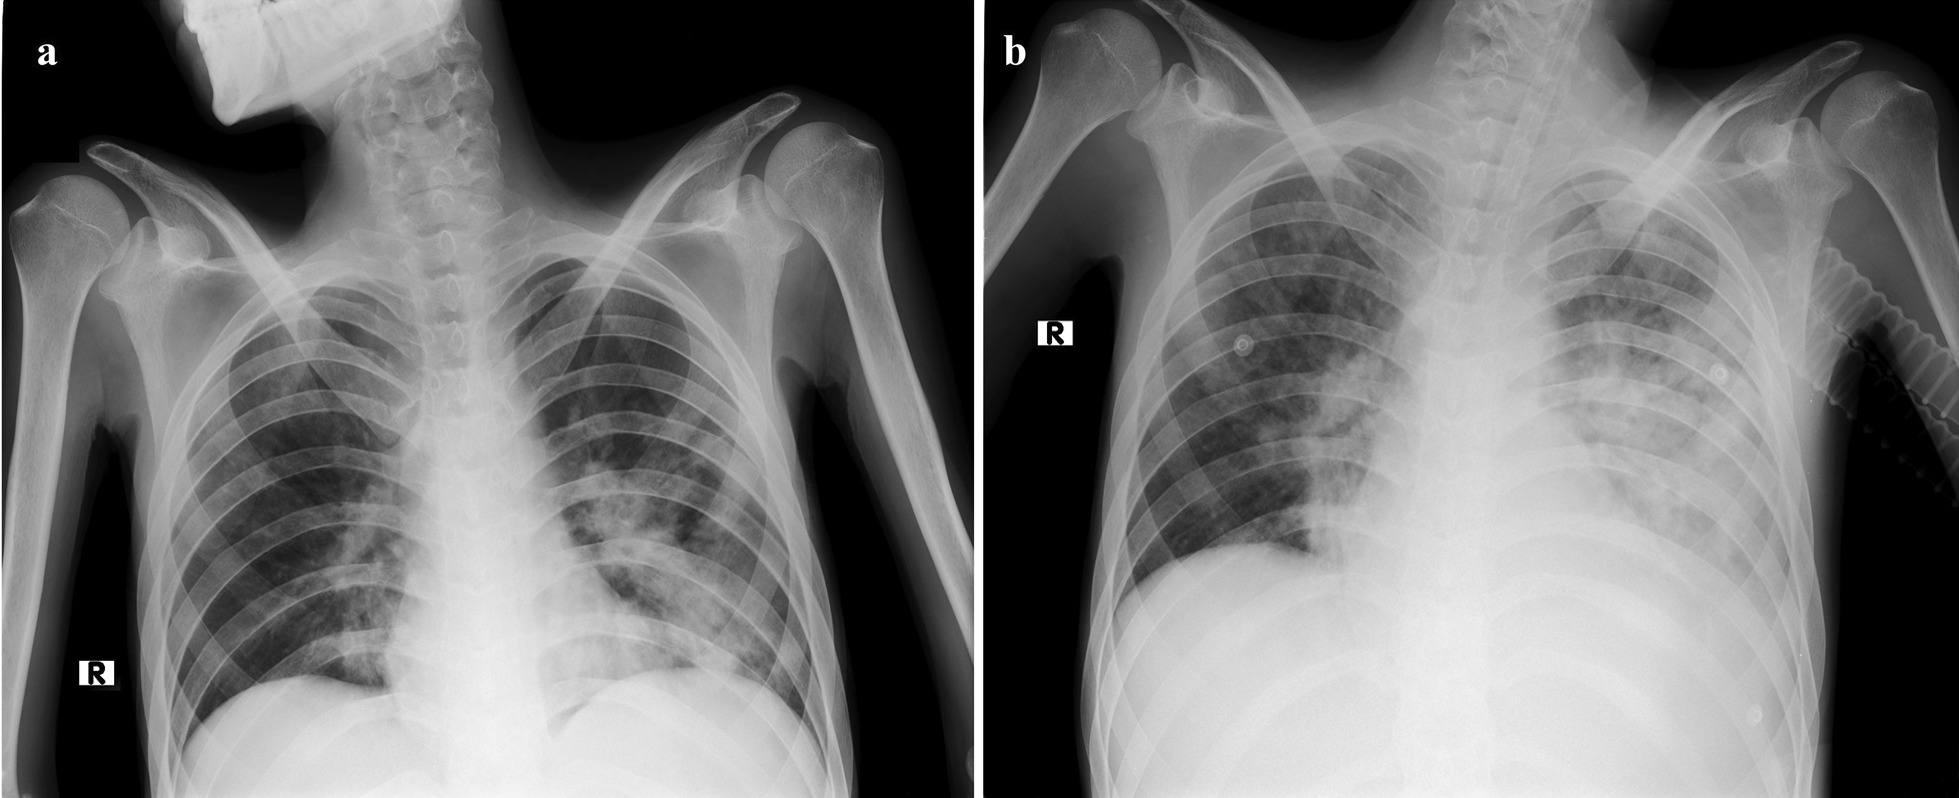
a, b The patient’s chest X-ray on his final admission leading to death. a Bilateral ground-glass opacities (more prominent in the left lung) on admission day; b rapid progressive parenchymal involvement of both lungs 4 days later
